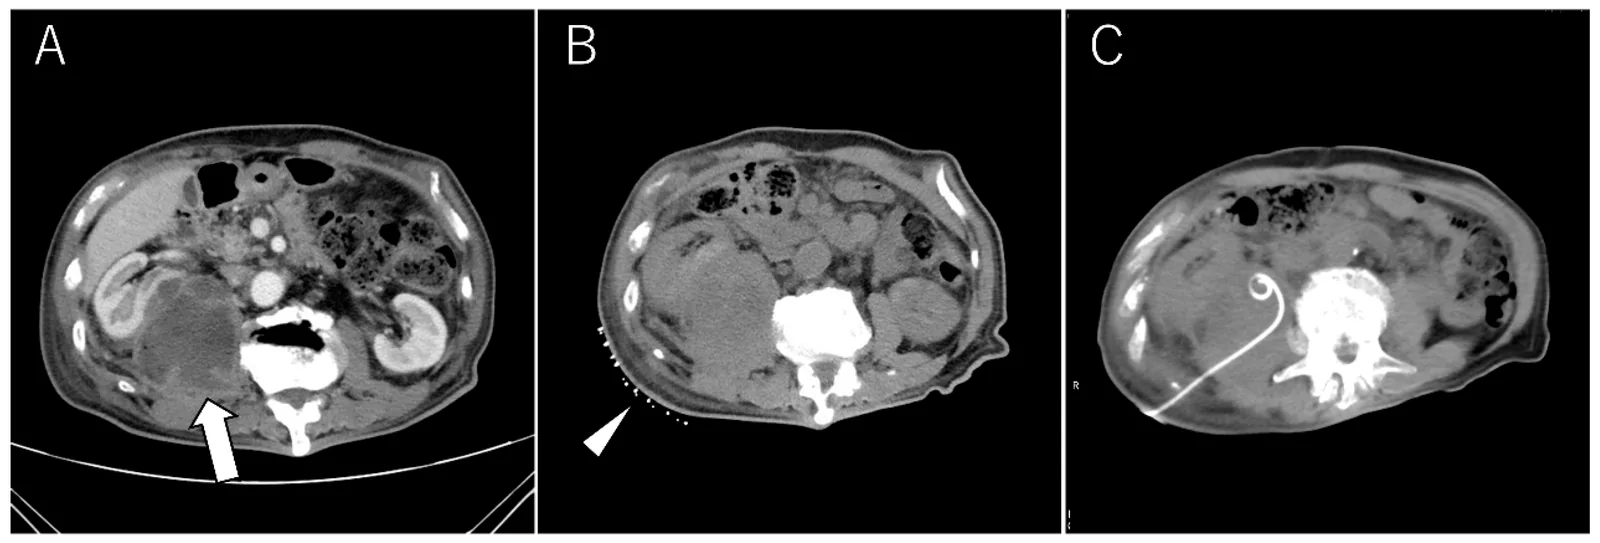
CT-guided drainage of a psoas abscess using a dorsal-space mat with a posterior approach. (A) Preprocedural contrast-enhanced CT image. A large abscess (arrow) is seen in the right psoas muscle. Due to severe back pain, drainage in the supine position was selected. (B) Positioning CT image during the procedure. The patient is placed supine on the dorsal-space mat, and the puncture site is marked (arrowhead). (C) CT image during drainage. The drainage tube was safely inserted via a posterior approach while the patient remained supine.
